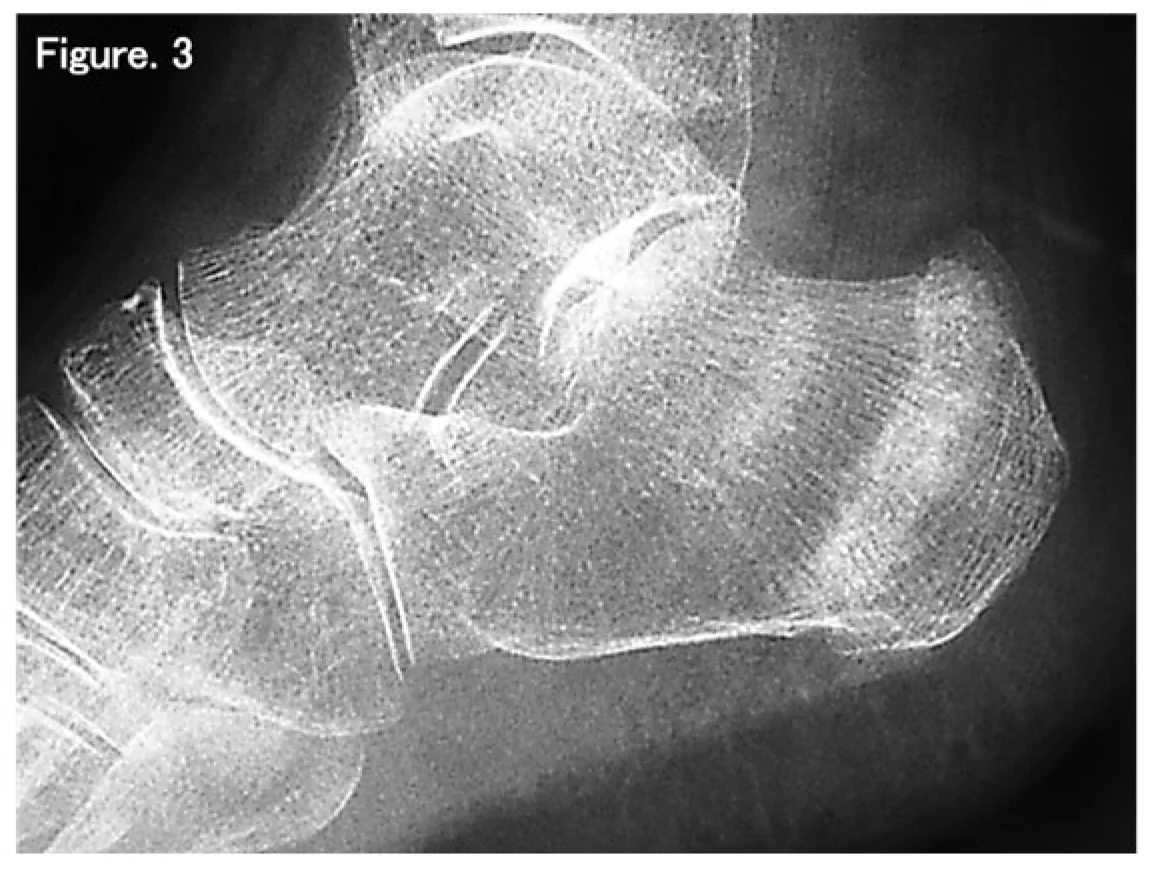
Initial radiograph of a 74-year-old woman who presented 5 weeks after symptom onset. Osteosclerotic change is already visible at the first visit.
